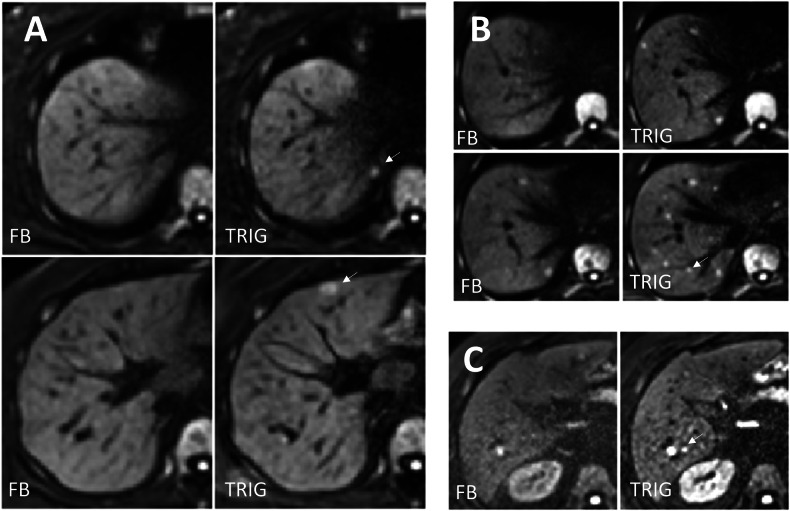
Liver DWI b800 images (A) of a 76-year-old patient with metastatic colorectal carcinoma, (B) of a 47-year-old patient with metastatic thyroid carcinoma, and (C) of a 60-year-old patient with metastatic neuroendocrine tumor. Different slices are shown for FB (left) and TRIG (right). The lesion that is only visible in TRIG images is marked with an arrow. All other lesions are recognizable with both techniques on neighboring layers, which are not shown. FB, free-breathing acquisition; TRIG, navigator-triggered acquisition
